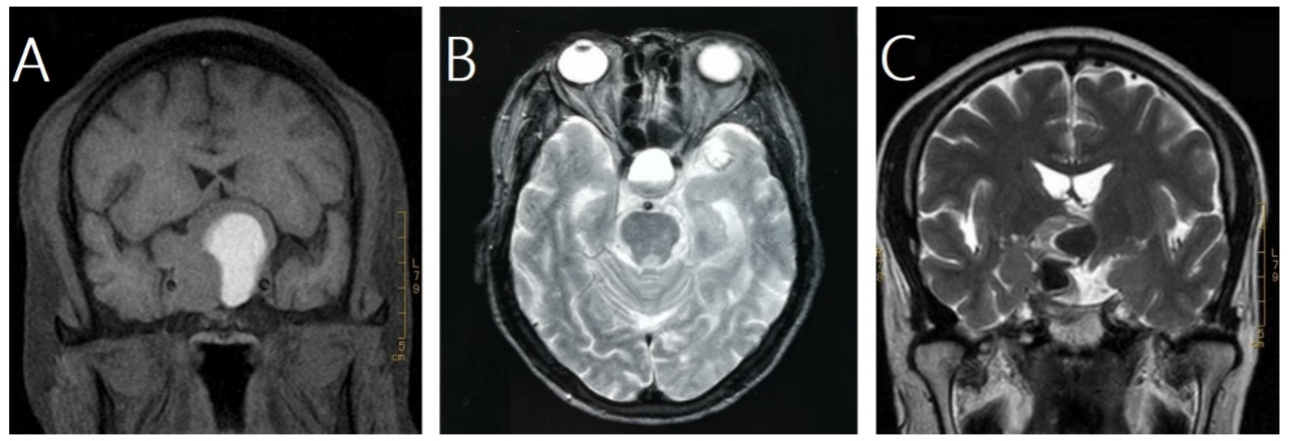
Magnetic resonance images of three cases of pituitary hemorrhage. A, a 43-year-old male with nonfunctioning pituitary adenoma (NFPA) presenting as a high intensity area on a coronal T1-weighted image. B, a 69-year-old male with NFPA showing the sign of a fluid-fluid level on an axial T2-weighted image. C, a 47-year-old male with NFPA presenting as a very low intensity area on a coronal T2-weighted image.
